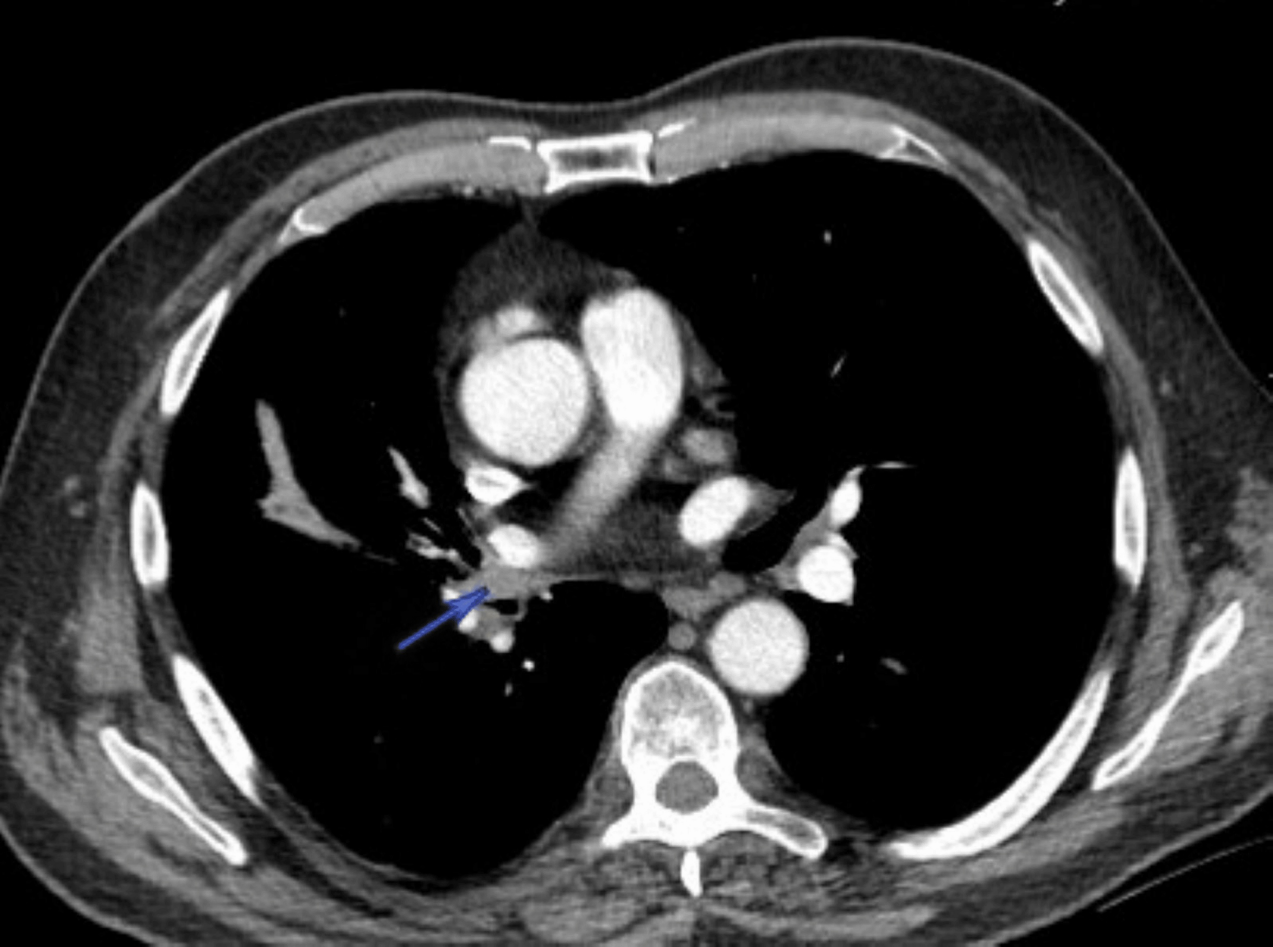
Computed tomography (CT) scan with intravenous (IV) contrast of the chest demonstrating a right hilar nodule (arrow) consistent with non-small cell lung cancer (NSCLC).
